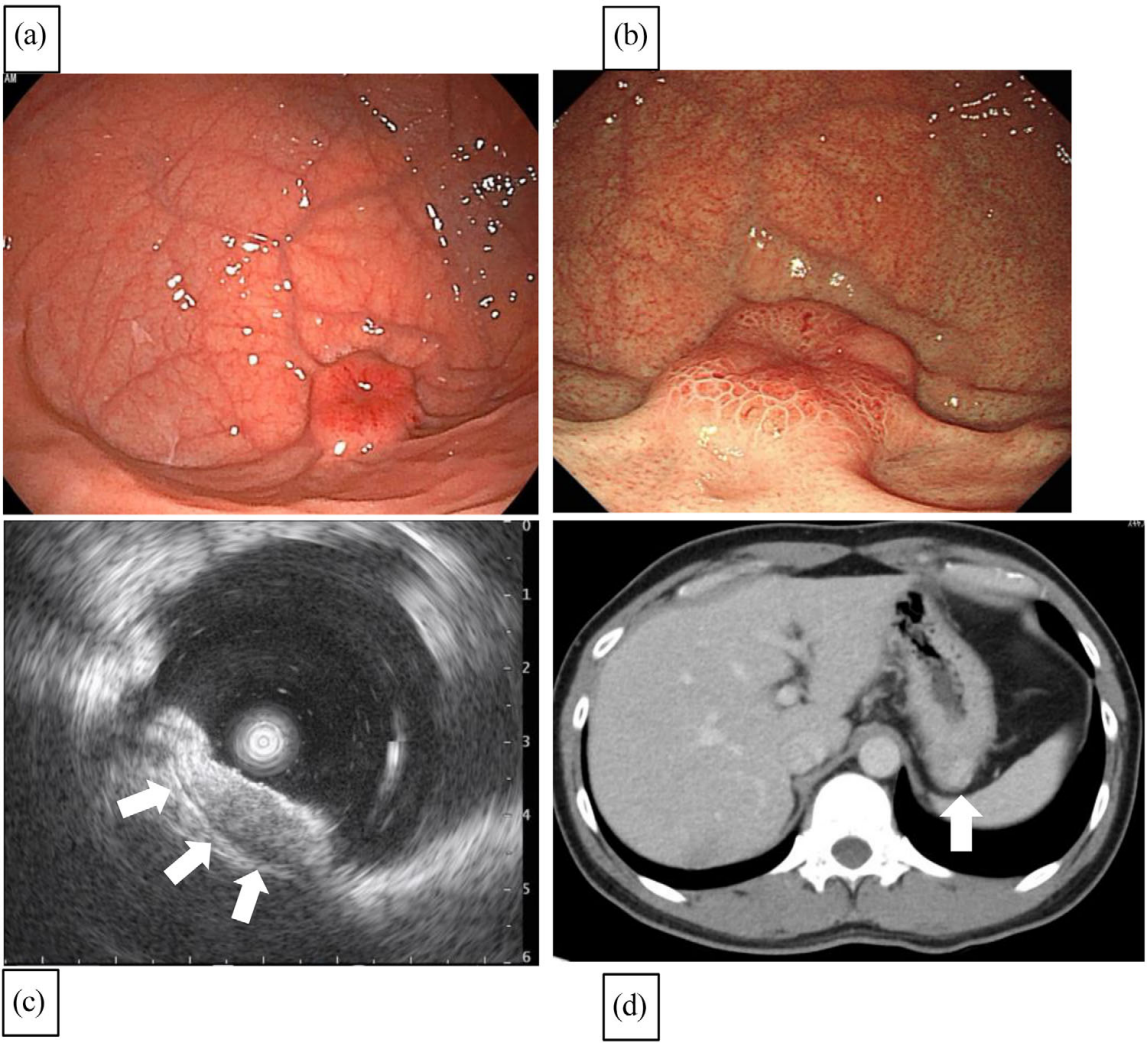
Esophagogastroduodenoscopy (EGD) and endoscopic ultrasonography (EUS) findings: (a) Previous physician's EGD findings reported a submucosal tumor‐like lesion in the fornix of the stomach. (b) EGD findings at our institution showed that the tumor was approximately 2 cm in length, and had increased in its thickness, with a reddish mucosal surface and central depression. (c) EUS revealed a tumor with a maximum diameter of approximately 20 mm with hypovascularity in the fourth layer and partially heterogeneous internal structures (white arrow). (d) Abdominal computed tomography showed no lymph nodes or distant metastases, just a tumor with a contrast effect in the same region of the gastric wall noted at endoscopy (white arrow).
